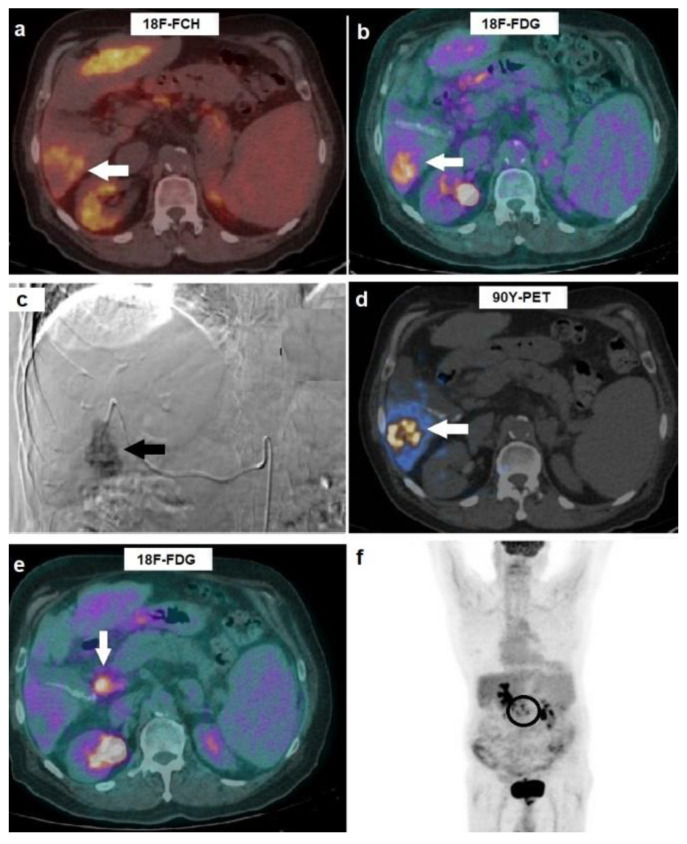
A 79-year-old man affected by HCV-related cirrhosis and HCC in the right hepatic lobe, previously treated with TACE and relapsed, referred to our center for ^90^Y-TARE. (a) Pre-treatment ^18^F-FCH PET/CT resulted negative for tracer incorporation in the HCC lesion located in the sixth hepatic segment (arrow). (b) Subsequently performed ^18^F-FDG PET/CT showed highly increased uptake (SUVmax 7.6) in HCC (arrow). (c) Pre-TARE angiogram, acquired after selective catheterization of the arterial branch for the sixth segment, clearly showed the hypervascularized lesion (arrow). (d) ^90^Y-PET, performed 4 h after ^90^Y-TARE, depicted selective microsphere accumulation in treated tumor (arrow). (e) On the 8-weeks post-treatment PET/CT scan, new-onset hypermetabolic pathological celiac adenopathies (arrow), in spite of complete regression of the treated HCC, were evident. (f) Maximum intensity projection ^18^F-FDG PET demonstrated well the metastatic localizations to abdominal nodes (circle).
